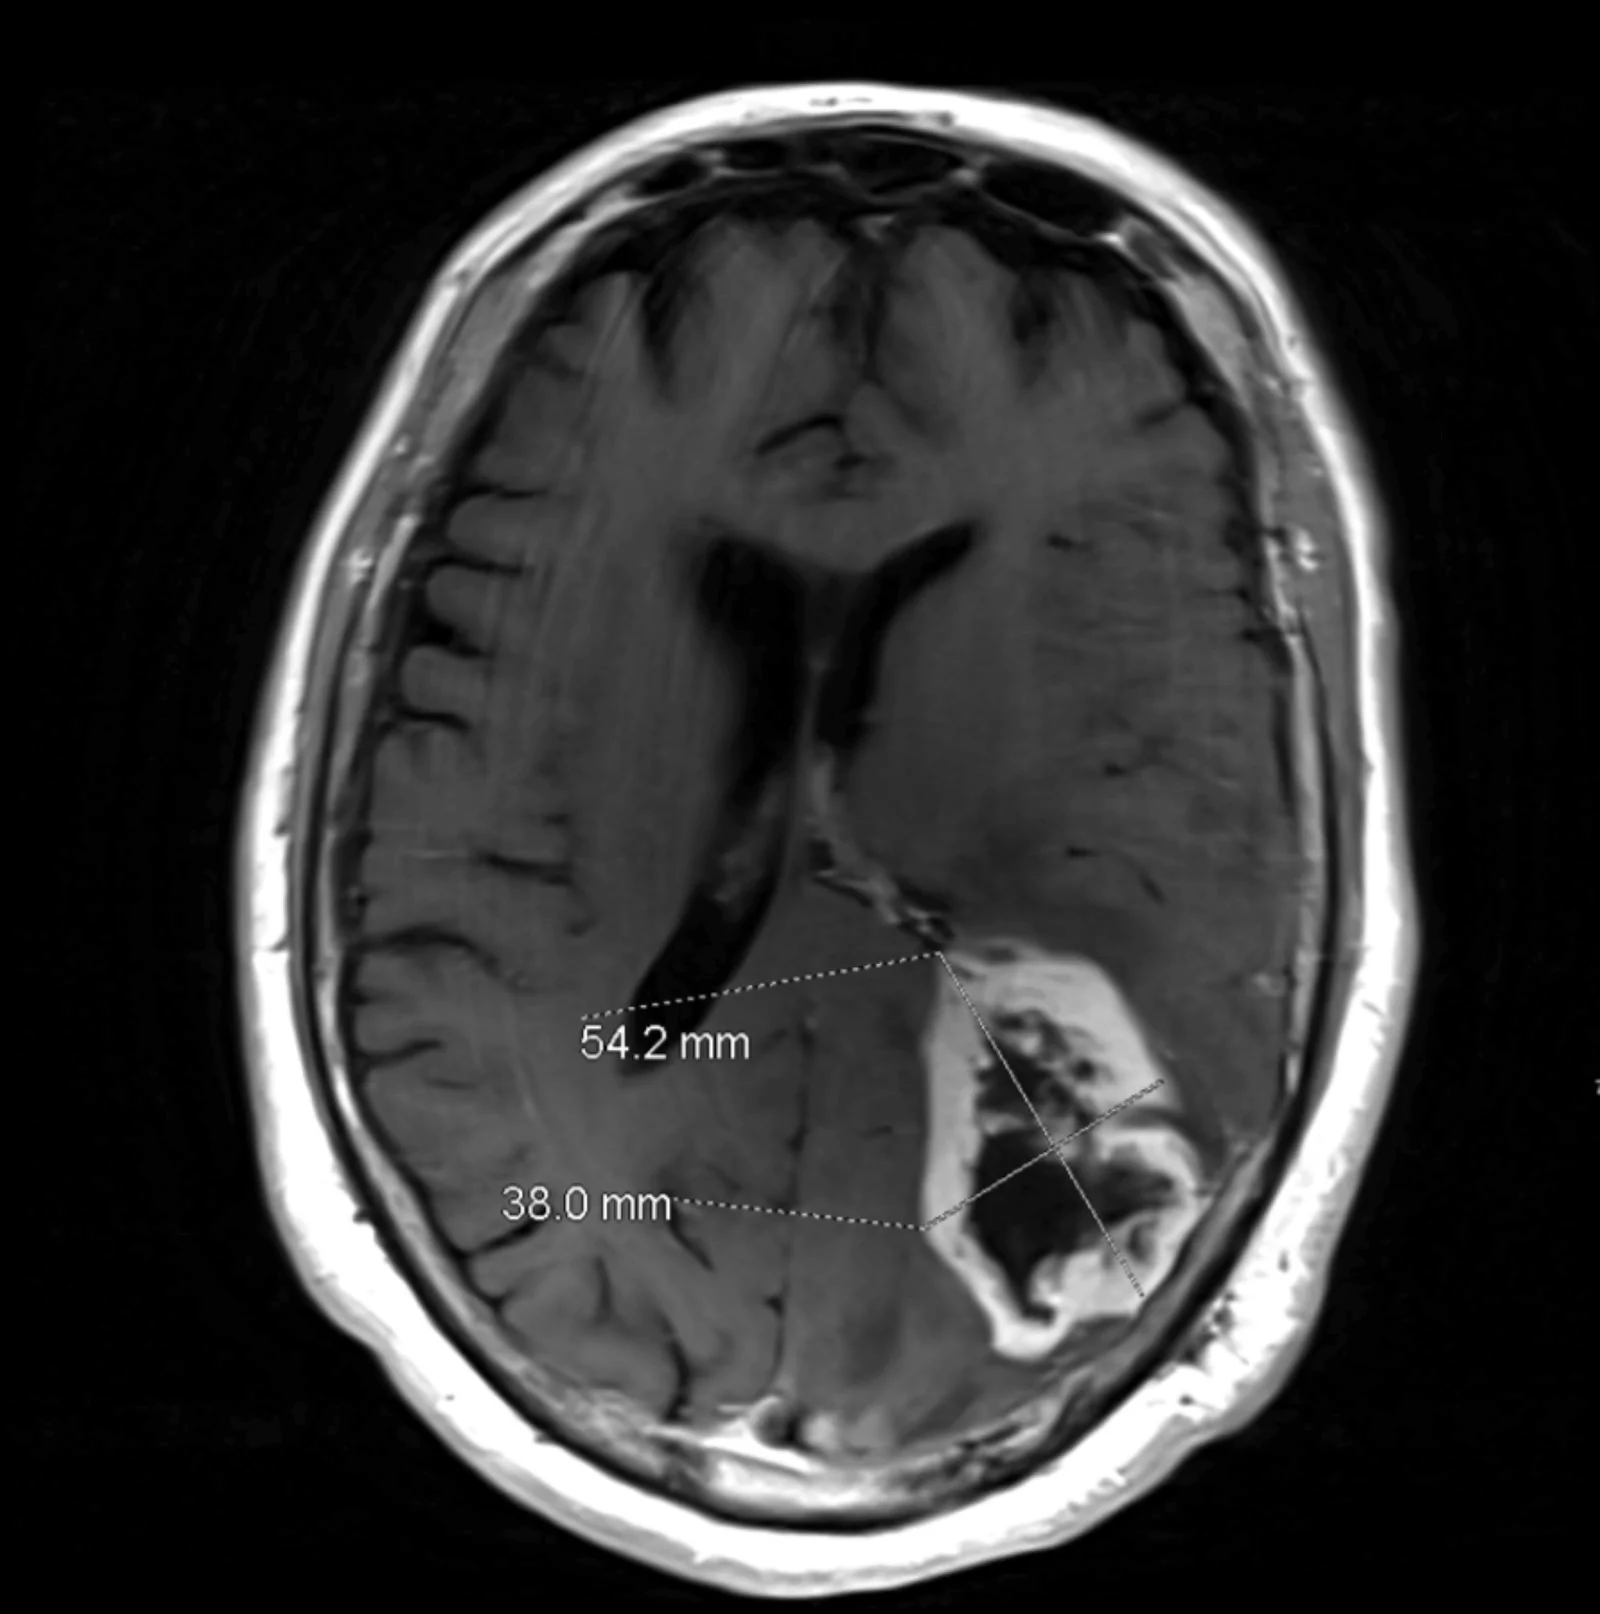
T1/FLAIR post-contrast brain MRI revealed a 5.4-cm heterogeneously enhancing mass in the left temporo-parieto-occipital region, with prominent perilesional edema, mild midline shift (7 mm), and internal areas of nonenhancing necrosis. FLAIR, fluid-attenuated inversion recovery; MRI, magnetic resonance imaging
